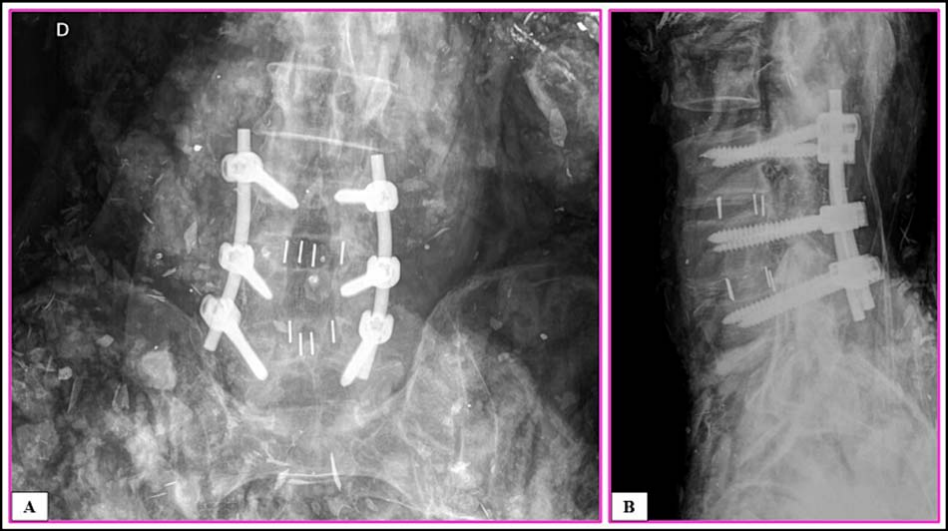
Postmortem X-ray of the lumbosacral spine in the anteroposterior (AP) (A) and lateral (B) views.
